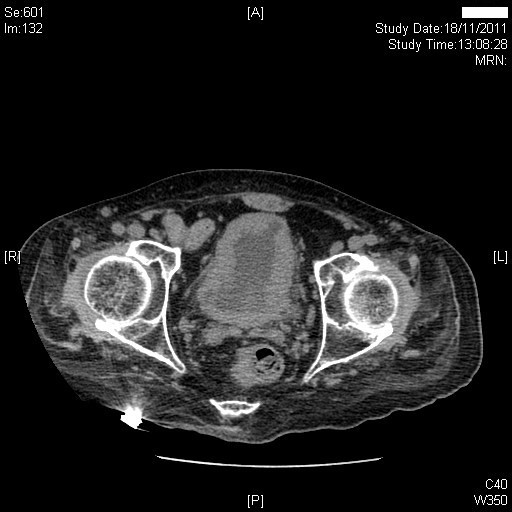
Computed tomography revealed marked circumferential thickening and trabeculation of the slightly contracted urinary bladder.
